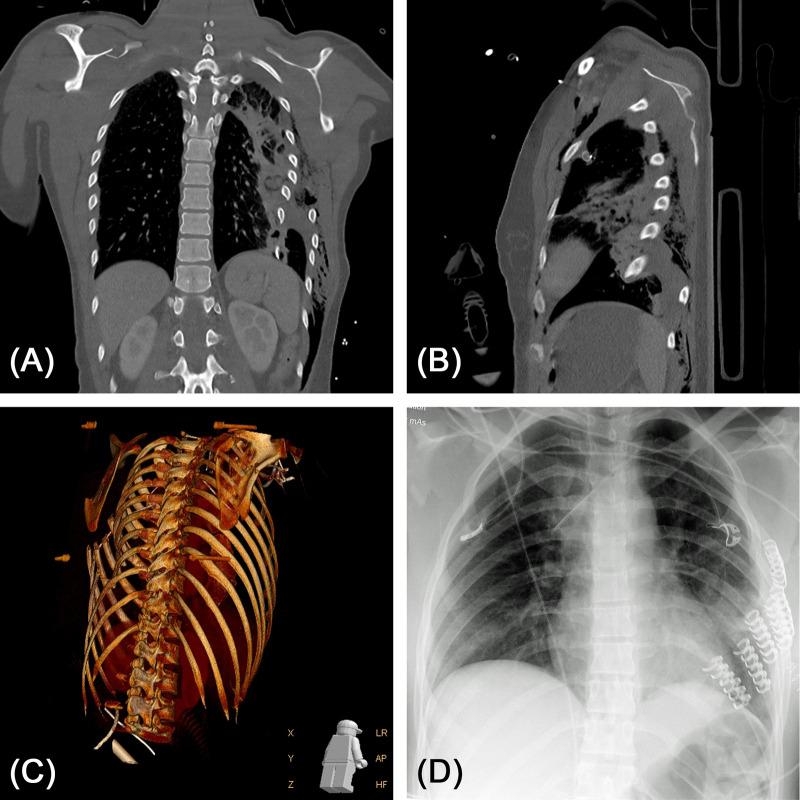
(A,B) 26-year-old female patient after suicidal train accident. Computed tomography (coronary and sagittal reconstructions are shown) revealed severely dislocated fractures of all ribs on the left side with laceration of the lung parenchyma and lung contusion. (C) 3D volume rendering of the CT dataset. (D) Post-operative chest X-ray after stabilization of costae 3 to 8. Laceration of the lung parenchyma was reconstructed by stapler-guided suture.
